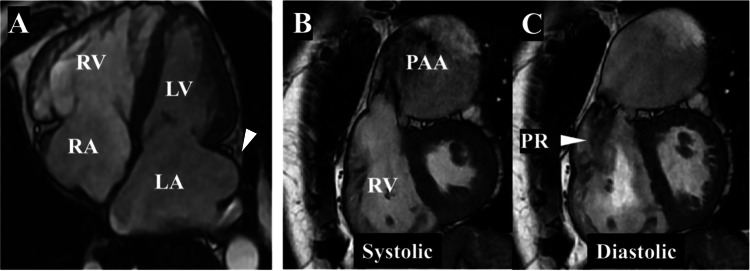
Preoperative MRI MRI revealed biatrial enlargement and right ventricular enlargement. The peripheral portion of the left atrium extended markedly beyond the posterior wall of the left ventricle (A, white arrow). A PAA and PR were also observed (B, C). LA: left atrium; LV: left ventricle; PAA: pulmonary artery aneurysm; PR: pulmonary regurgitation; RA: right atrium; RV: right ventricle
